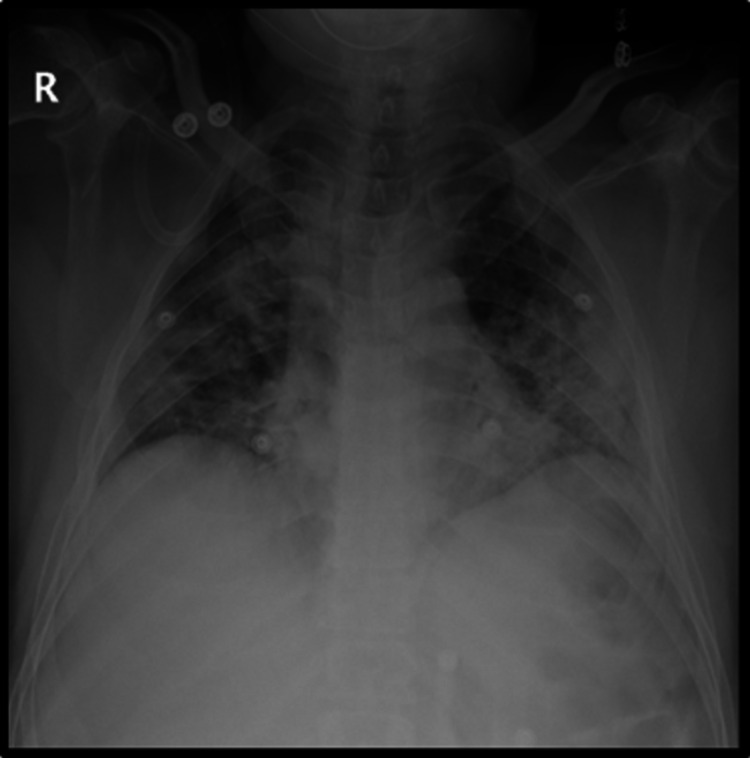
Chest X-ray revealed bilateral non-homogenous opacities at middle and lower lung zones along with blunted both costophrenic angles.
